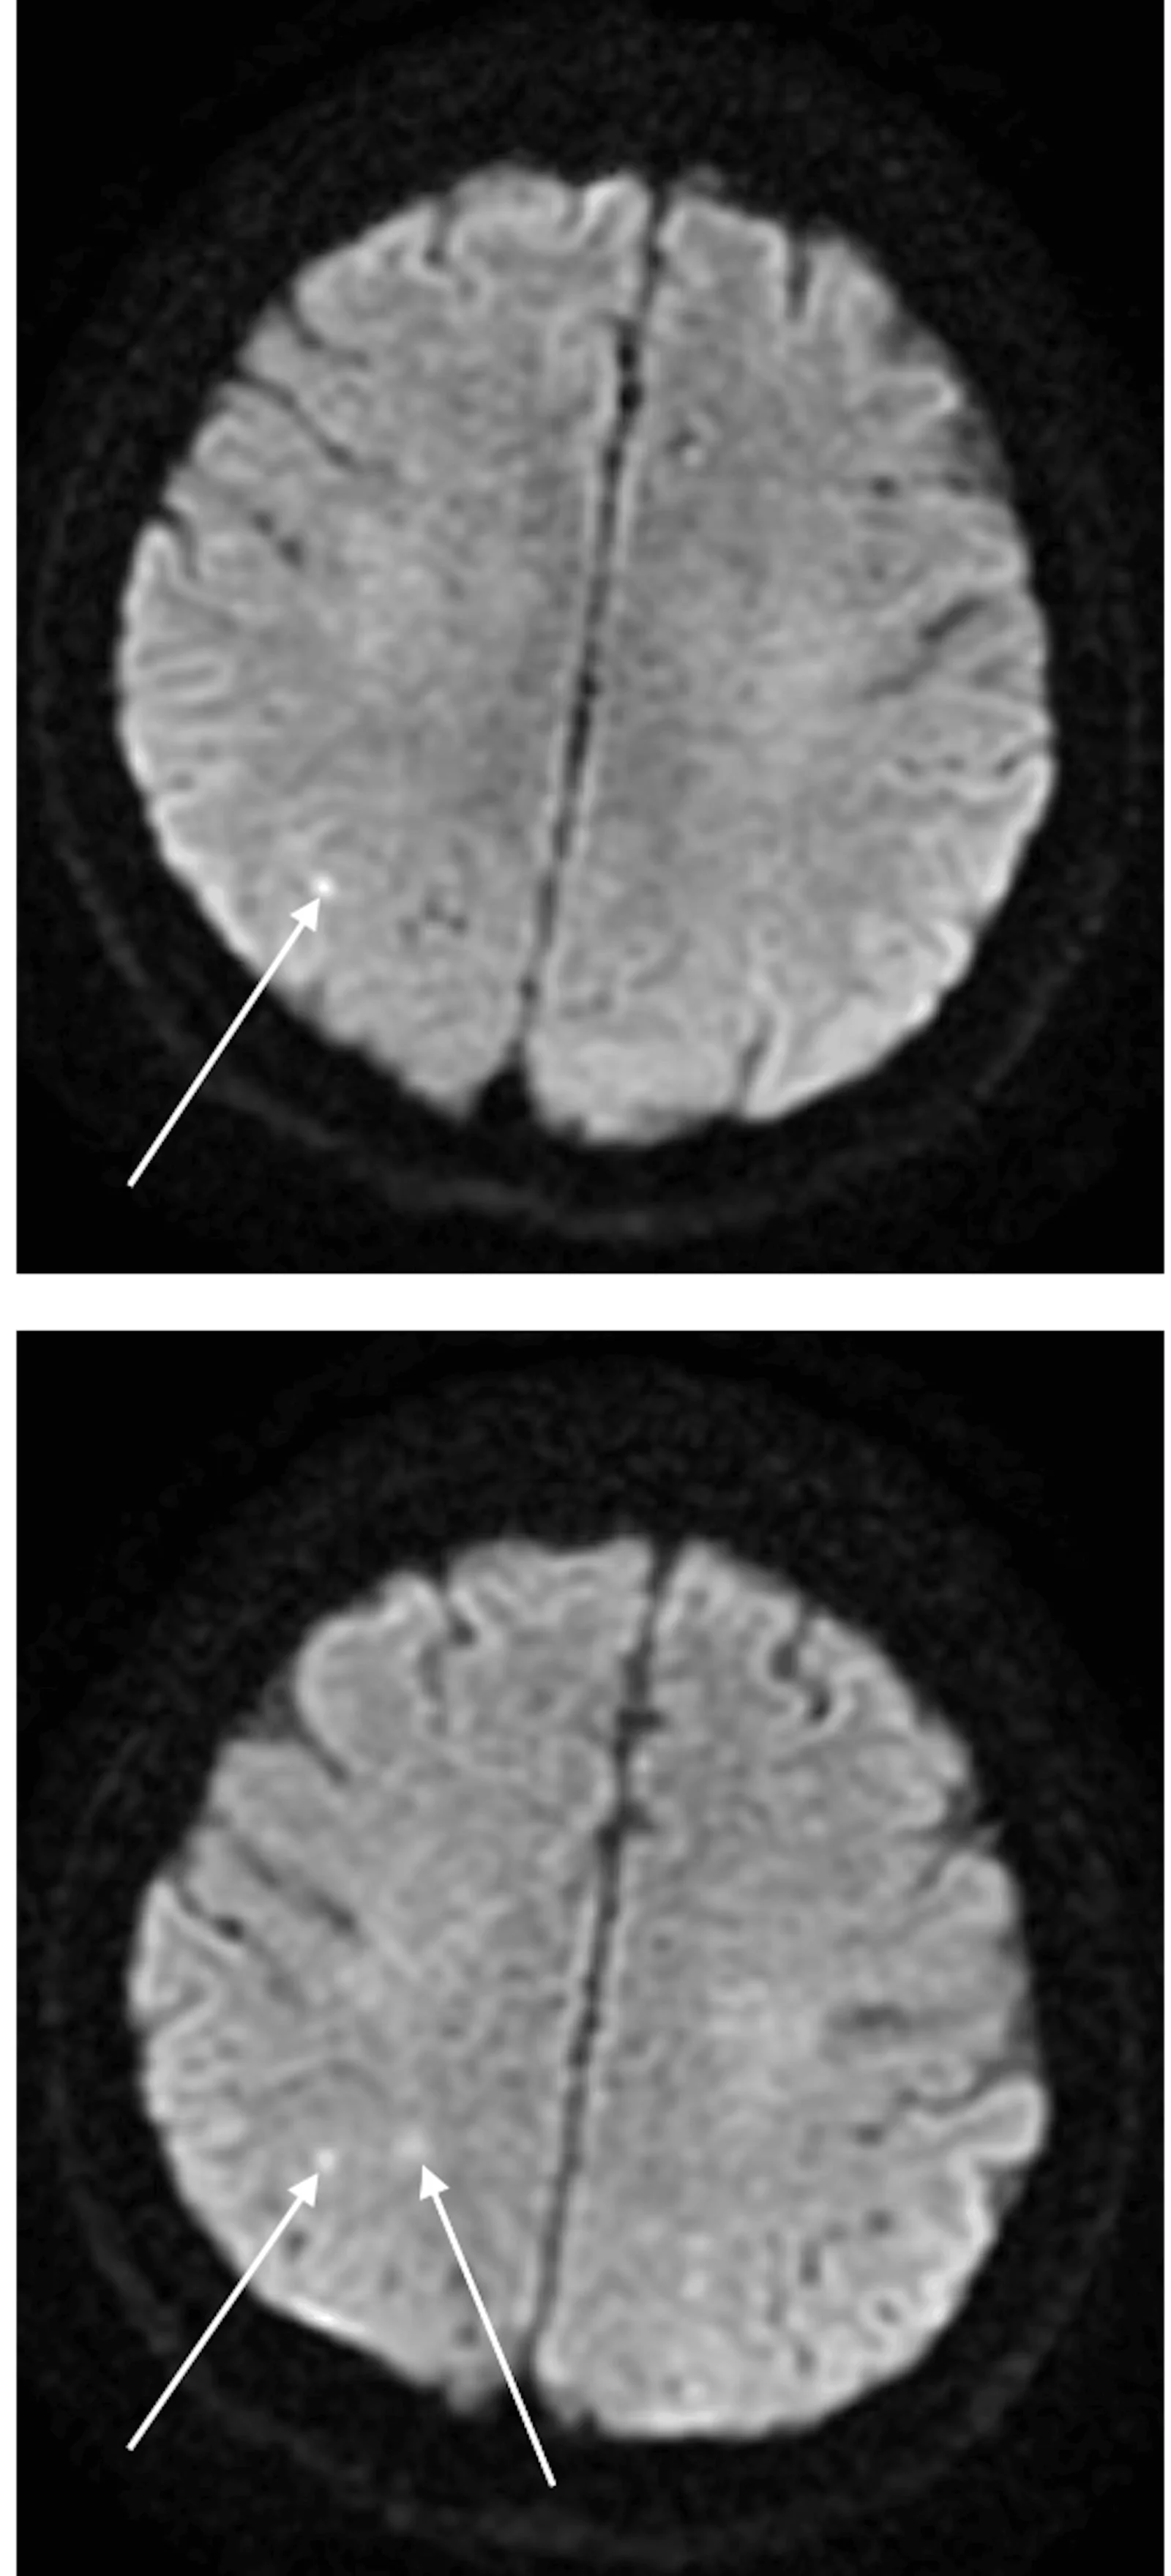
Brain magnetic resonance imaging demonstrating punctate acute infarcts in the right parietal lobe (arrows), consistent with microvascular ischemia in thrombotic thrombocytopenic purpura
